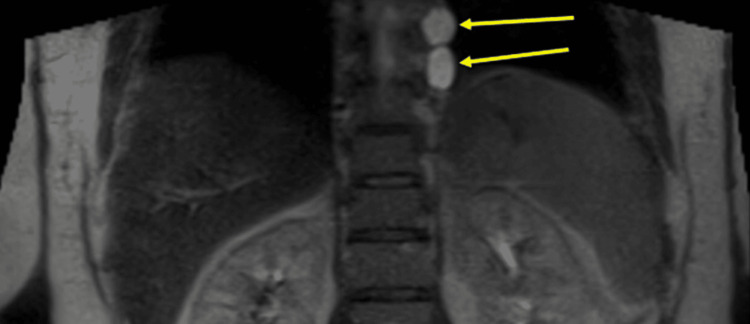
Full-body MRI showing nerve sheath tumors on the left from levels T4 to T8 T2 hyperintense paraspinal non-enhancing lesions on the left from levels T4-T8. The largest is at the T7 level and measures 2.0 x 1.4 cm, previously 2.2 x 1.9 cm.
